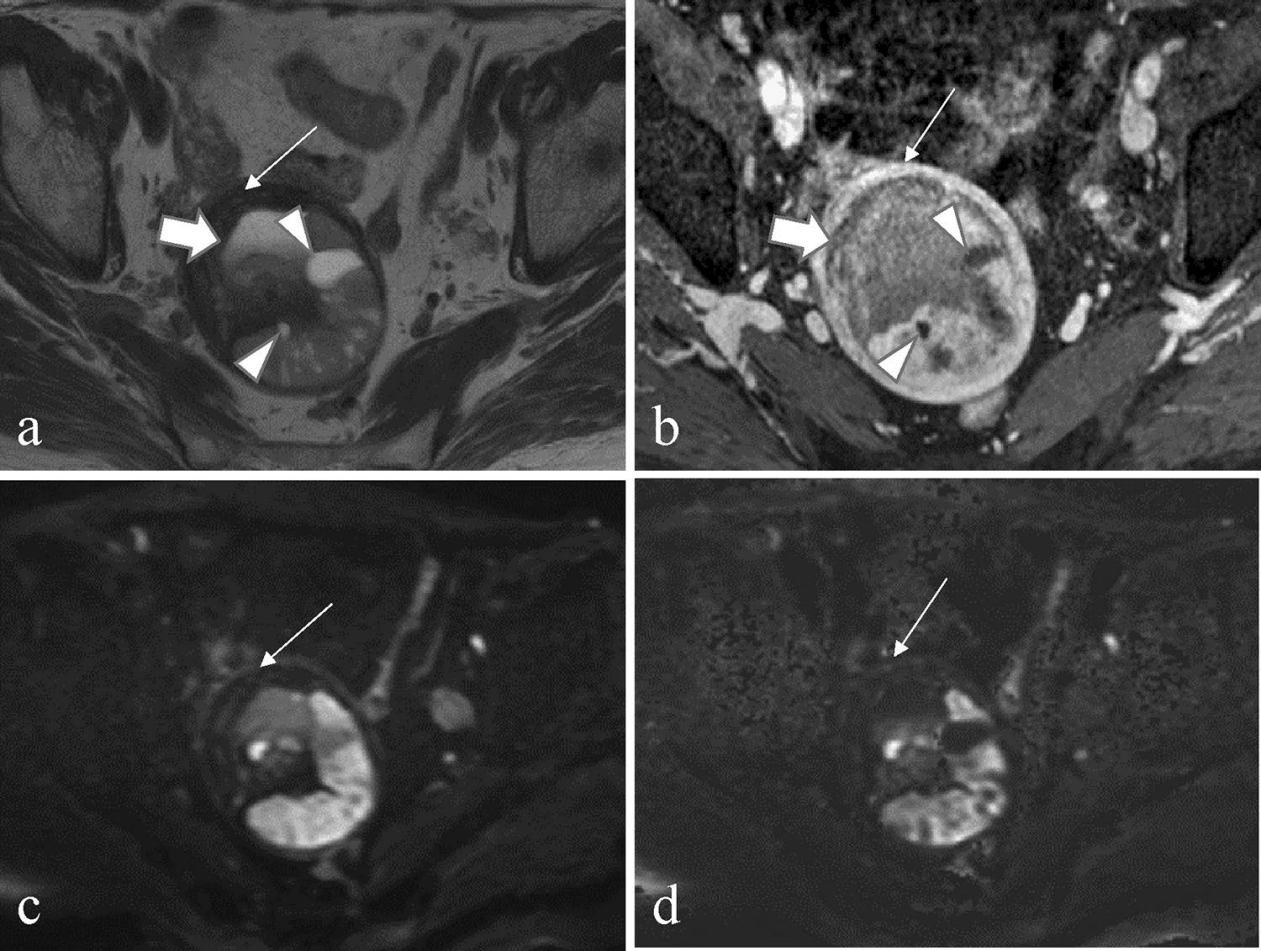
A 76-year-old woman with endometrial stromal sarcoma without abnormal vaginal bleeding. a The axial T2-weighted image and b axial contrast-enhanced T1-weighted image with fat suppression show a tumor, located mainly in the myometrium, with hemorrhagic necrosis (arrow: necrosis and T2 dark area) and cyst (arrowhead). c On an axial diffusion-weighted image with a *b*-value of 1000 s/mm^2^ and d an axial computed diffusion-weighted image with a *b*-value of 2000s/mm^2^, the solid component of endometrial stromal sarcoma appears to have a higher signal than the myometrium (thin arrow). The lesion shows restricted diffusion, and the mean apparent diffusion coefficient value is 0.86 × 10^−3^ mm^2^/s
